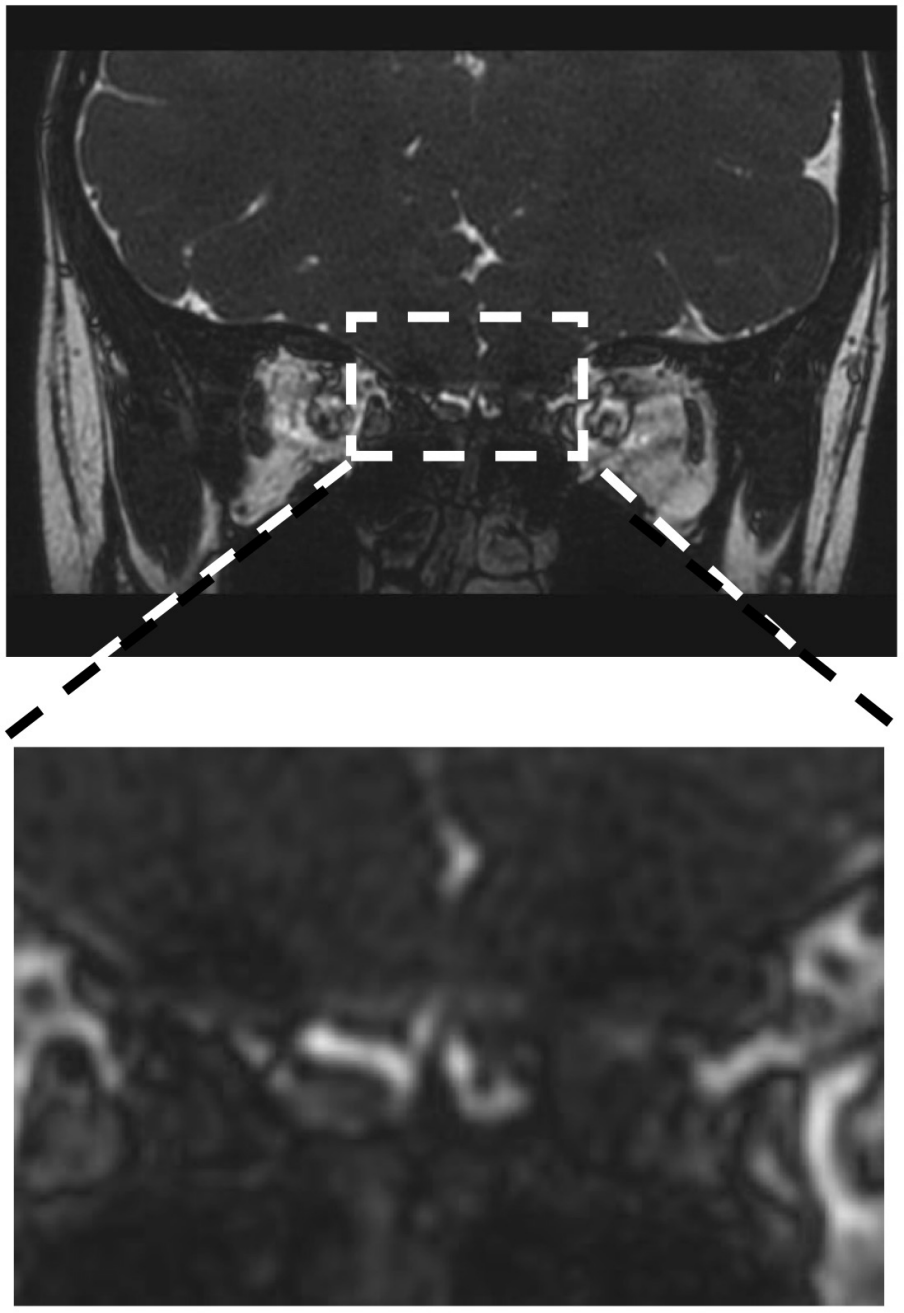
Clinical findings of the patient. Magnetic resonance imaging showed hypoplasia of the left olfactory groove and olfactory bulb.
